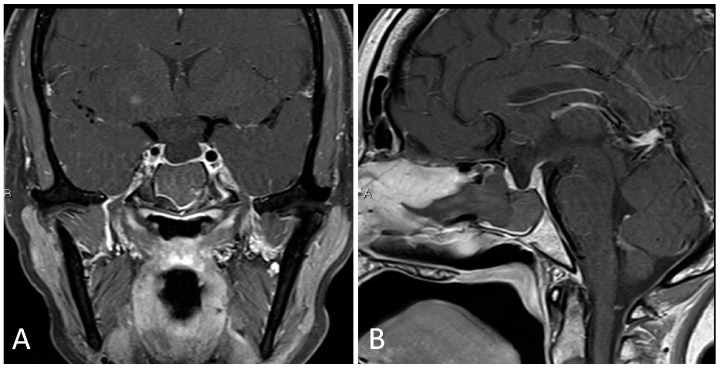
Postoperative imaging consistent with gross total resection of Rathke’s cleft cyst. (A) Coronal T1 post-contrast MRI. (B) Sagittal T1 post-contrast MRI.
